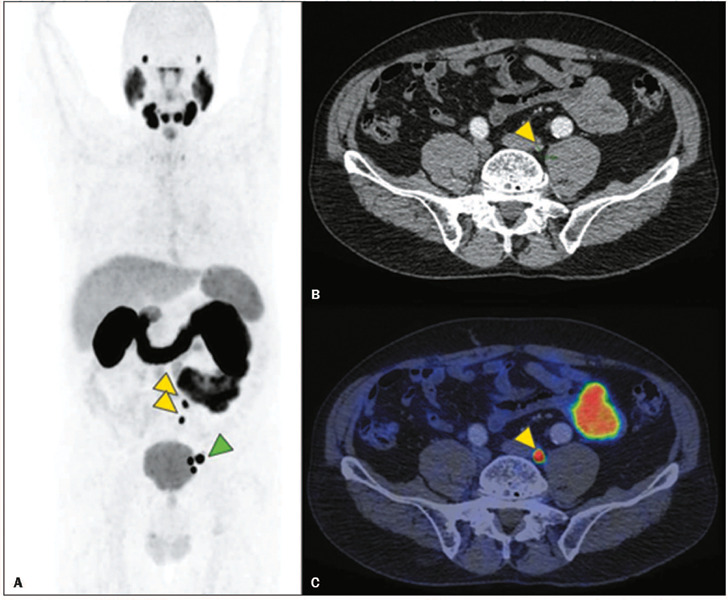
Example of PSMA PET/CT findings that have an impact on therapeutic management.
